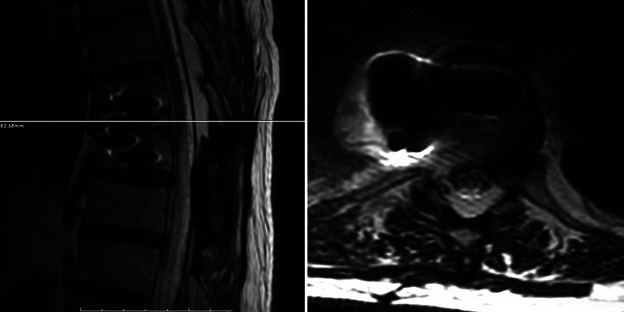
Postoperative focused T2-weighted sagittal (left) and corresponding axial (right) magnetic resonance (MR) images show thorough decompression of the cord.
